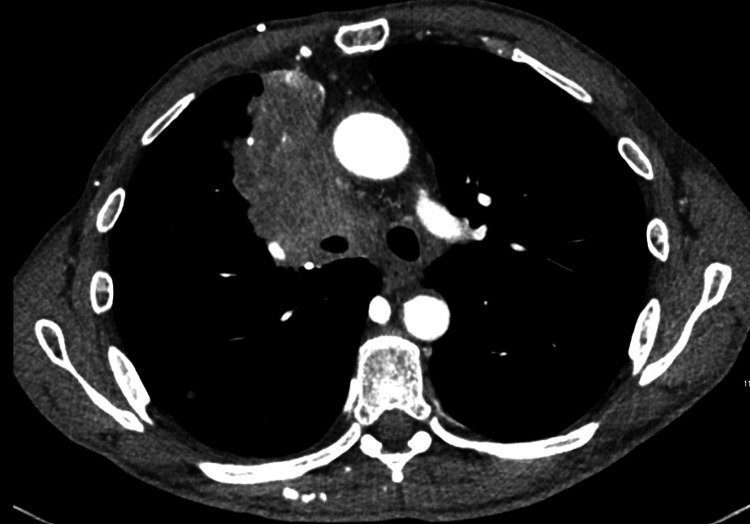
Contrast-enhanced CT thorax shows a pulmonary mass originating from the right upper lobe, with direct invasion into the mediastinum and occlusion of the superior vena cava
